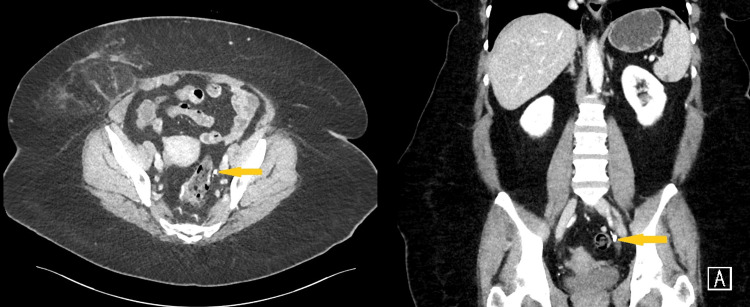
Combined axial and coronal CT imaging demonstrating a left-sided mid-ureteral calculus Axial (left) and coronal (right) contrast-enhanced CT images of the abdomen and pelvis. The yellow arrows indicate a hyperdense calculus located in the mid-portion of the left ureter at the level of the pelvic brim, a common site of ureteral narrowing.
